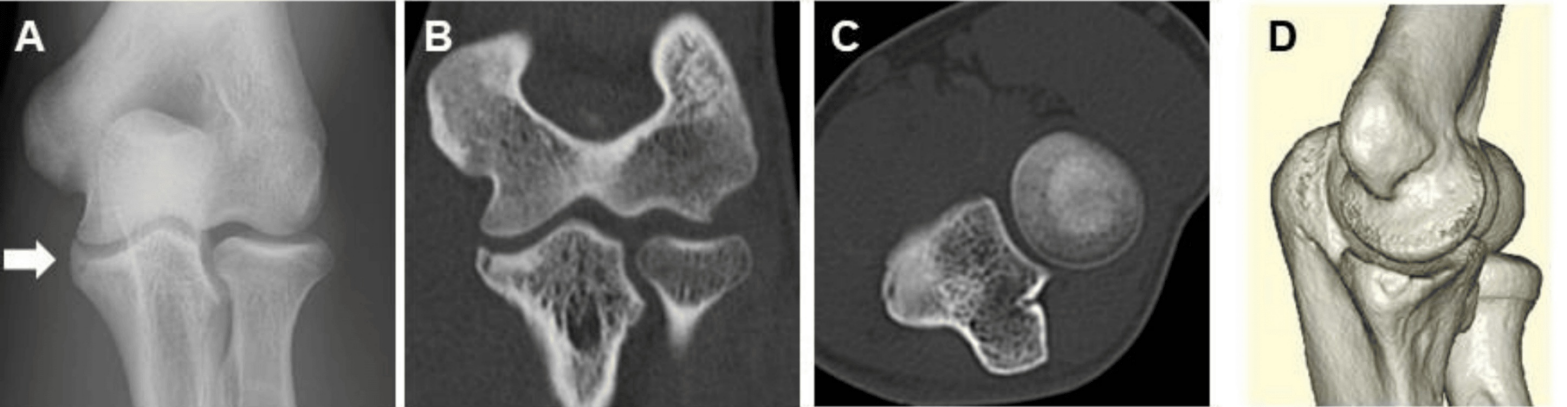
Preoperative radiographic and CT findings of case 2 A. Initial anteroposterior radiograph showing a small bony fragment at the ulnar sublime tubercle (white arrow). B. Coronal CT image. C. Axial CT image. D. Three-dimensional CT reconstruction.
